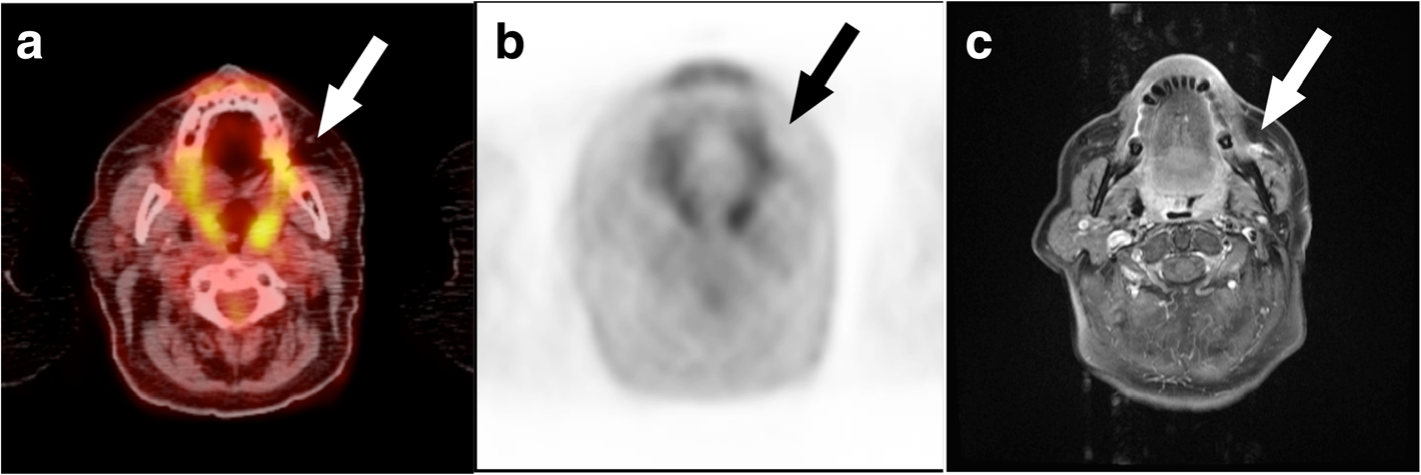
No tumour recurrence: PET/CT imaging was true-negative, indistinctive finding on MRI. Patient after multiple resections of recurrent tumours of a left buccal adenoid cystic carcinoma. A hyperintense lesion is seen on the contrast-enhanced T1-weighted MR image (c) (arrow), but there was no pathological finding on the fused PET/CT (a) and PET image (b). The follow-up confirmed no recurrence
